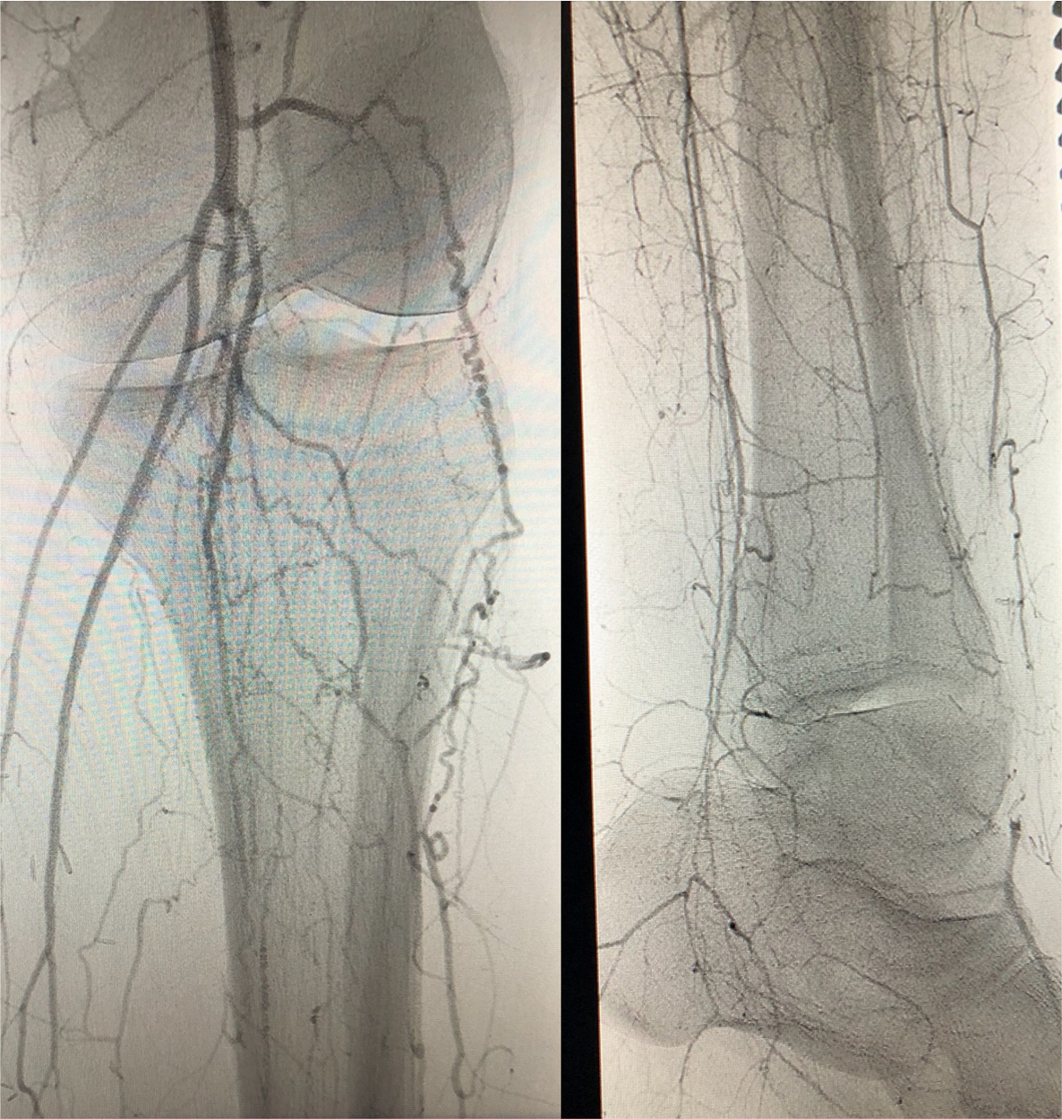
Right Lower Extremity CT Angiogram April 2017 Left: Right distal superficial femoral and popliteal artery collateral circulation development. Right: Right lower extremity collateral circulation to a level of dorsalis pedis artery.
